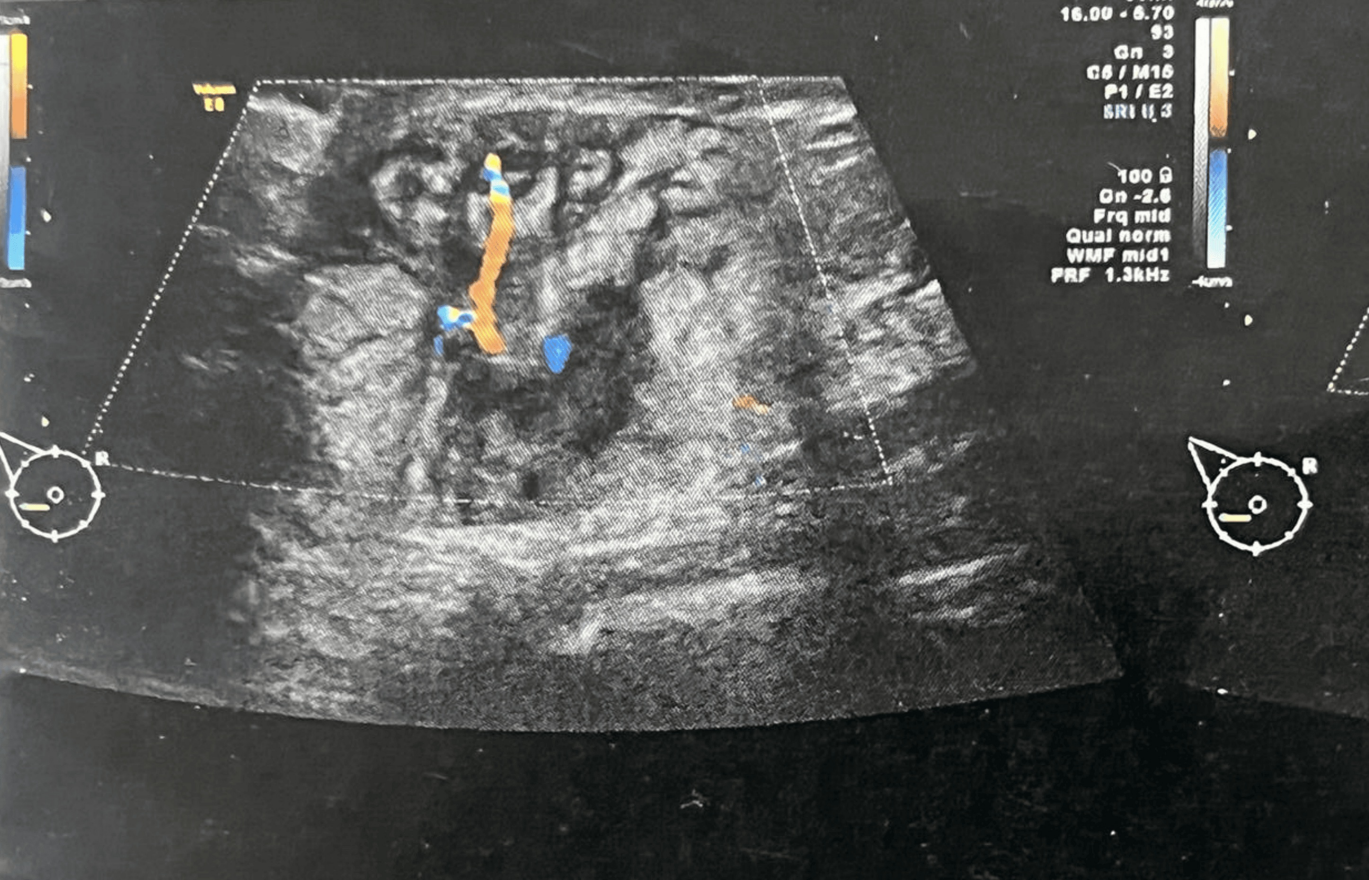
Ultrasound of the right breast Ultrasound imaging demonstrated a hypoechoic, heterogeneous, and hypervascular lesion with irregular margins measuring 3.7 × 2.7 cm. The marked vascularity and absence of posterior acoustic shadowing were imaging features supportive of lymphoma.
